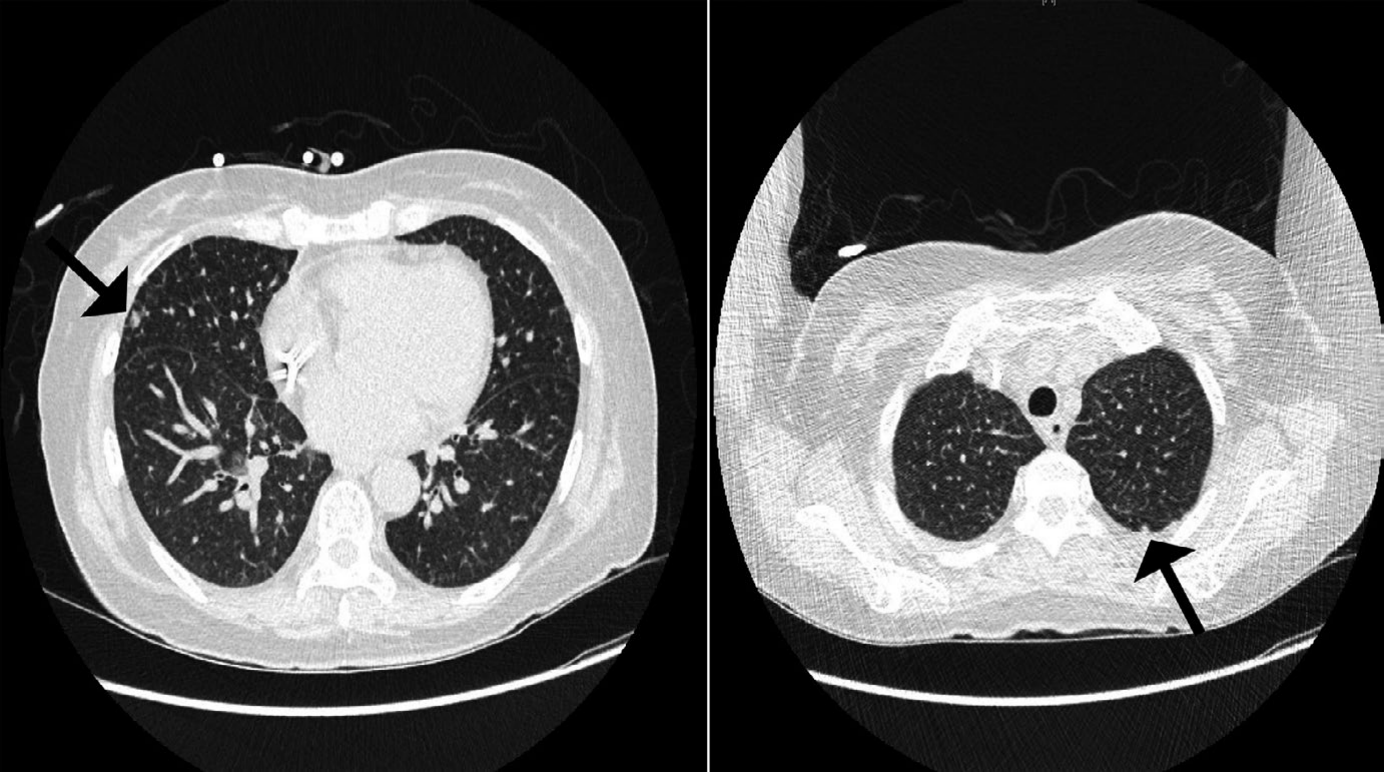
Chest non‐contrast computed tomography showed tiny nodules in both lungs. Arrows indicate tiny nodules in the right middle lobe and left upper lobe of the lungs, suggestive of disseminated tuberculosis infection
